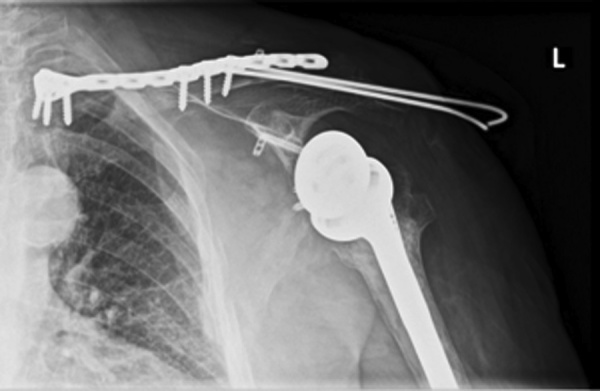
Radiograph of the left shoulder one month post open reduction and internal fixation of bipolar clavicular fracture, showing good bone healing with stable construct and satisfactory alignment, along with an intact reverse shoulder prosthesis.
